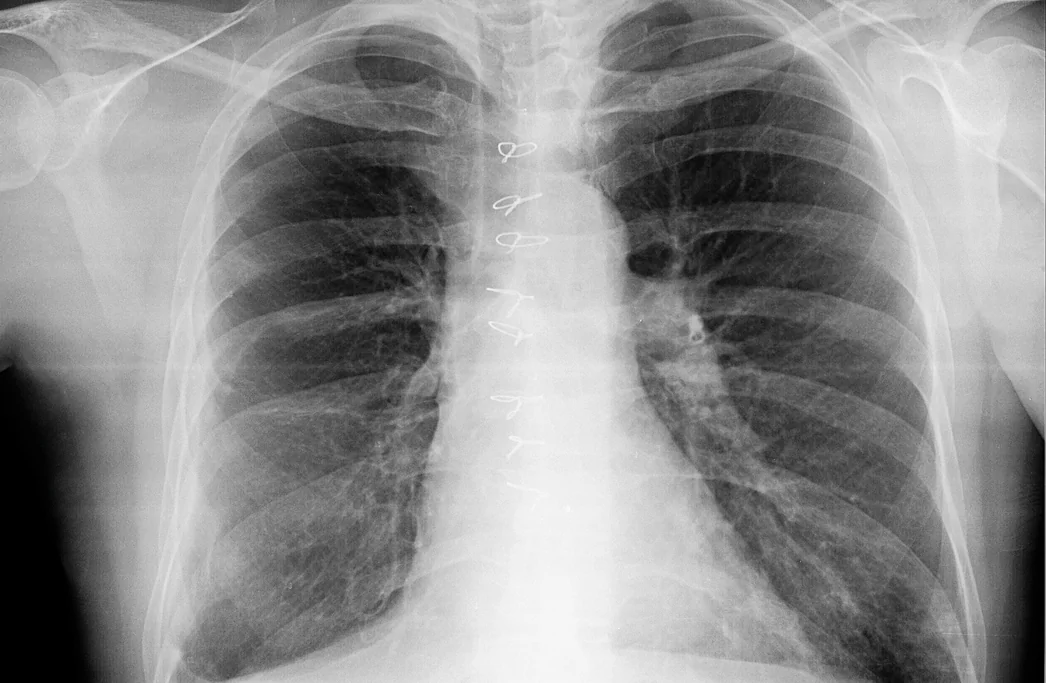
Chest Radiography Chest radiograph demonstrated poststernotomy changes consistent with prior pulmonary endarterectomy, with mild prominence of perihilar pulmonary vasculature and blunting of the right costophrenic angle suggestive of a small pleural effusion. No significant cardiomegaly was observed.
